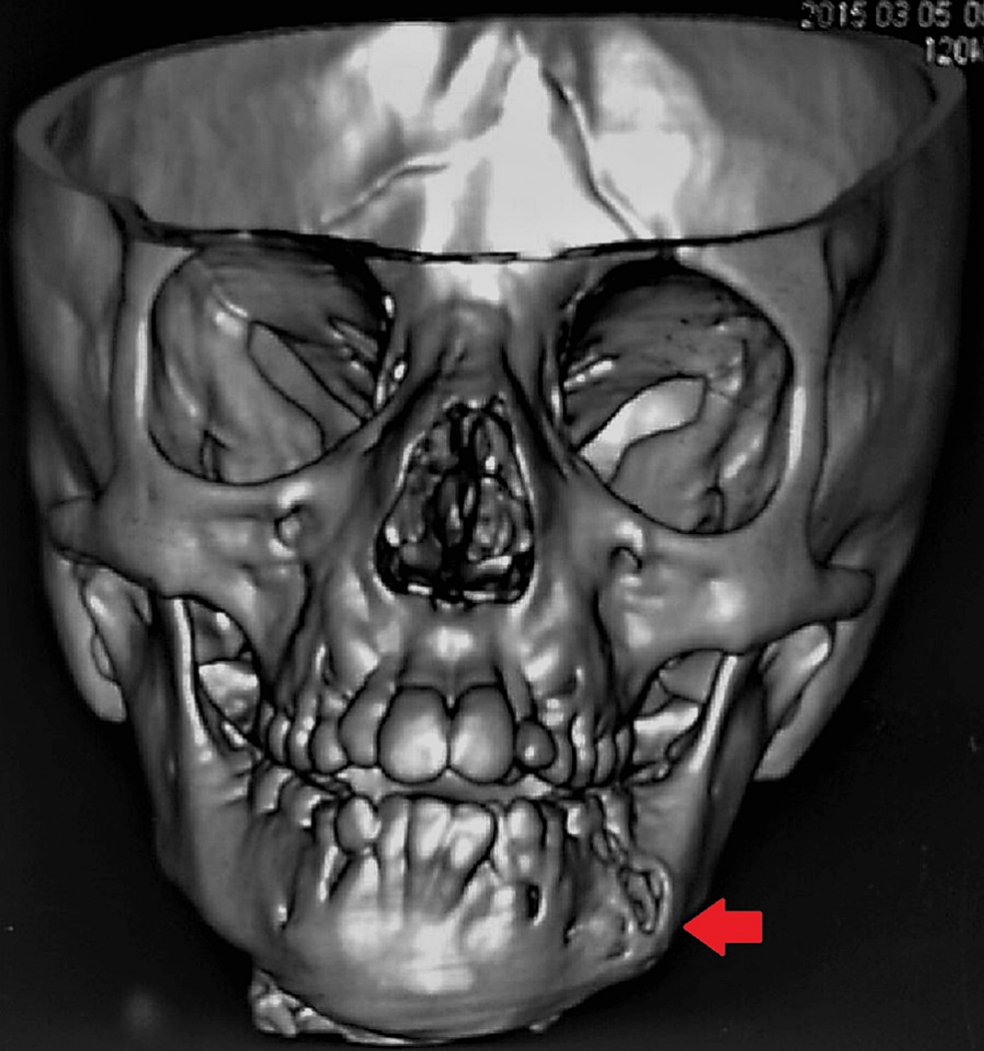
Computed tomography scan of facial bone showing a lytic lesion in the left mandibular region with buccal cortical expansion and perforation
